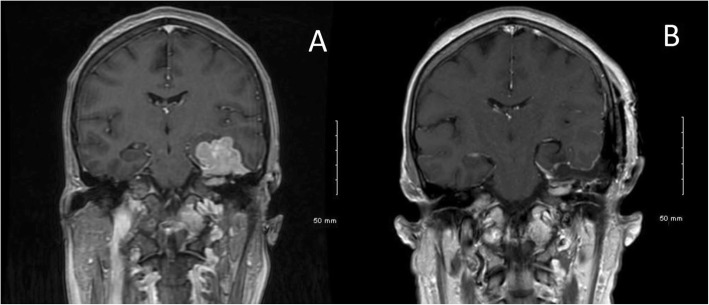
Representative T1-weighted post-contrast images preoperatively (a) and postoperatively (b). Pre-operative (a) and post-operative (b) coronal MRI, T1-weighted-post contrast. A lobulated, homogenously enhancing lesion is seen to be arising from the dura of the middle fossa and tentorium and compressing the temporal lobe. Gross total resection is noted in the post-operative image
